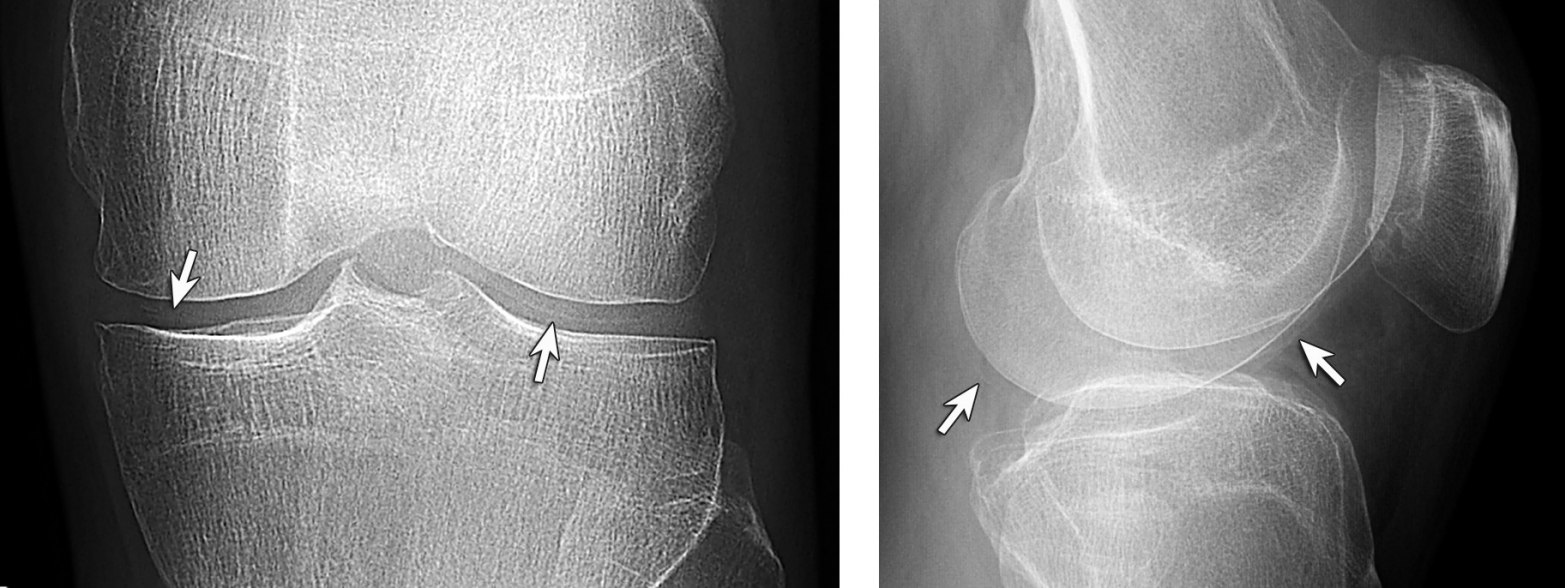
40-year-old man with gout. Knee radiographs (A, anteroposterior and B, lateral) show linear cartilage icing (arrows). No chondrocalcinosis is present on the images.
